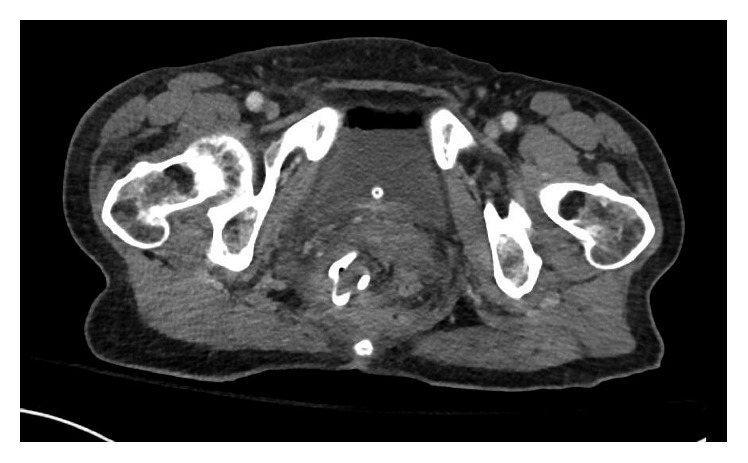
CT on day 15 after drainage demonstrating resolution of abscess with transanal drain in place.
